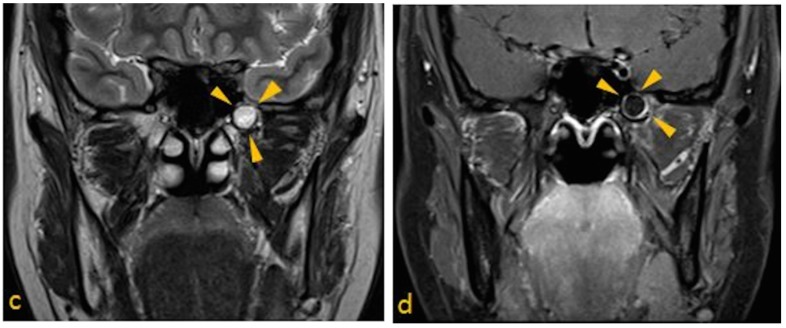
MRI features of arrested pneumatization of sphenoid sinus. (a) T1 paracoronal pre-contrast image; (b) T1 paracoronal post contrast image; (c) T2 paracoronal post gadolinium images, show hyperintense lesion in the left pterygoid process without enhancement; and (d) T1 paracoronal post-FS, shows homogeneous loss of signal, consistent with fat.
